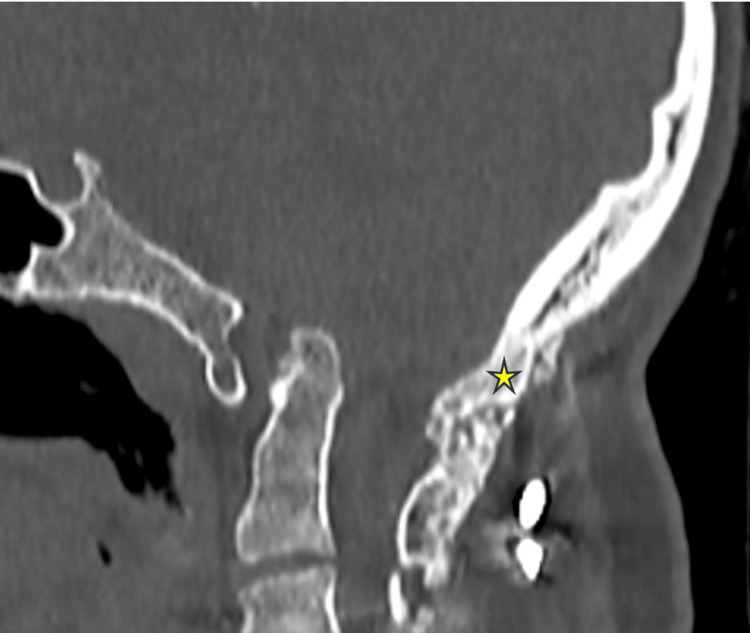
A follow-up CT scan image of cranio-vertebral junction with the star mark showing solid fusion mass with thick trabeculae formation from occiput to C2 lamina.
